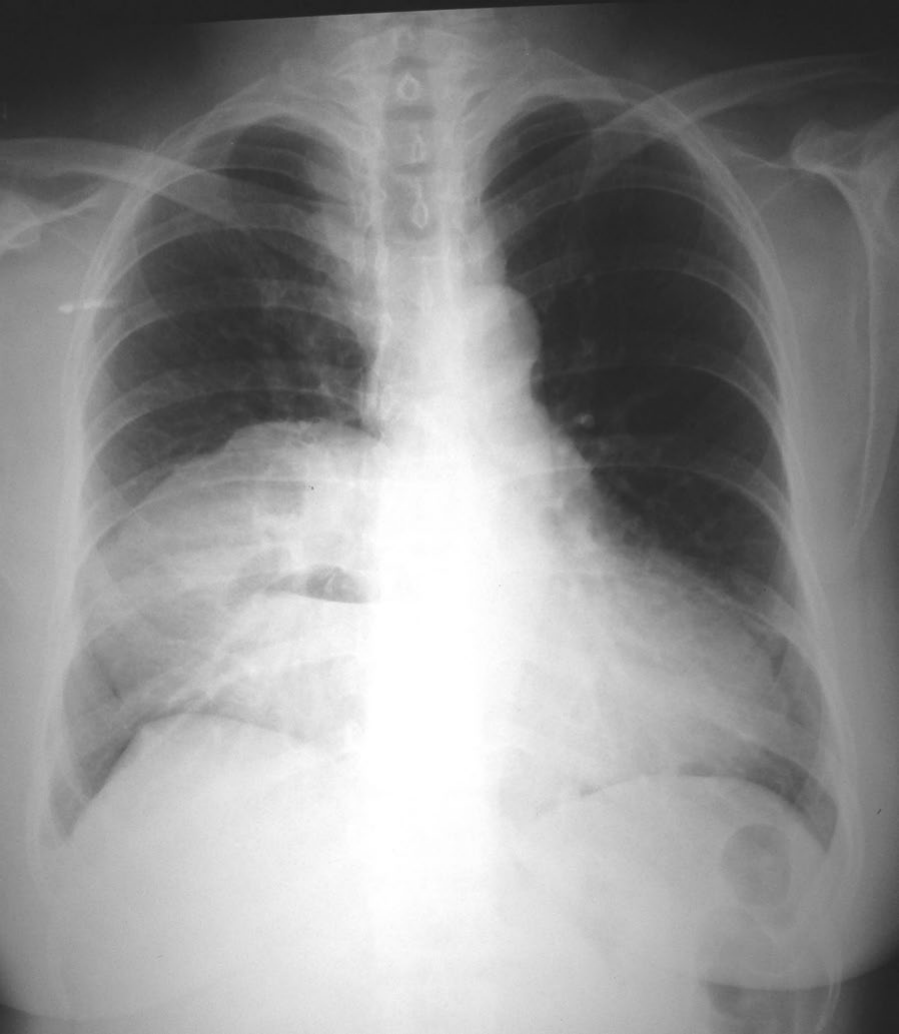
Preoperative chest radiograph of patient 1 showing right paracardiac shadow.
